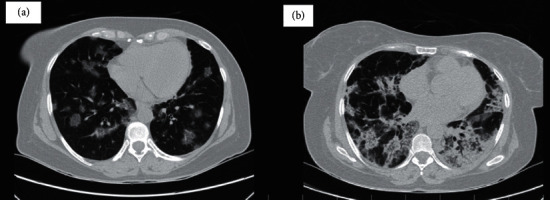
Spiral chest CT scans imaging: (a) diagnostic chest CT scan showing bilateral multifocal, dominantly peripheral, patchy ground-glass opacities suggestive of COVID-19 pneumonia. (b) Follow-up chest CT scan after 18 days showing notable progression in bilateral alveolar opacities with coarse reticular pattern (pulmonary fibrosis) in comparison with previous CT.
